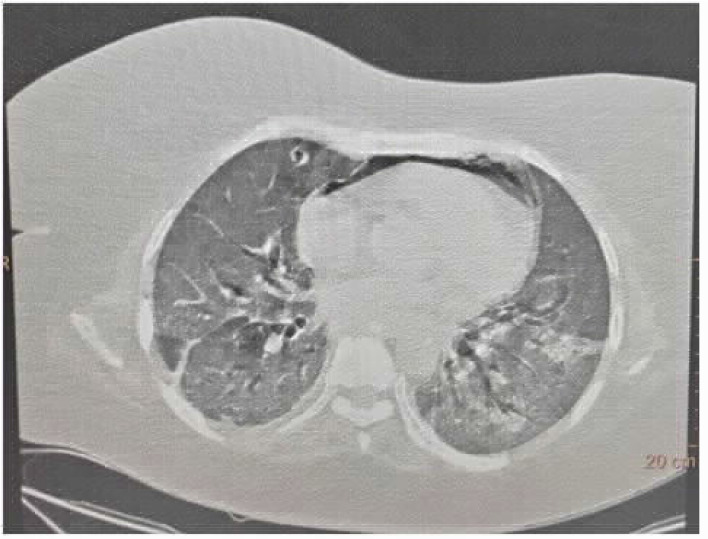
CASE 12: pneumopericardium on CT image
